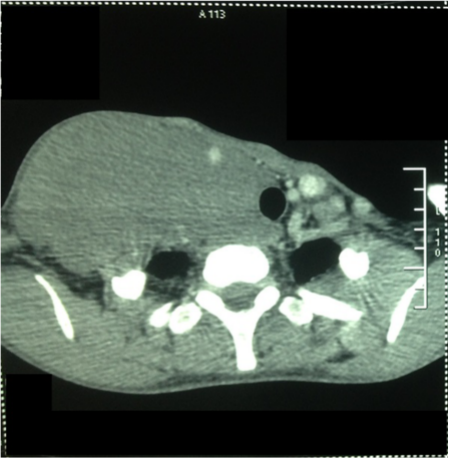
Axial computed tomographic scan showing the patient’s cervical mass, which was displacing the larynx, the trachea, and the thyroid gland outwardly
